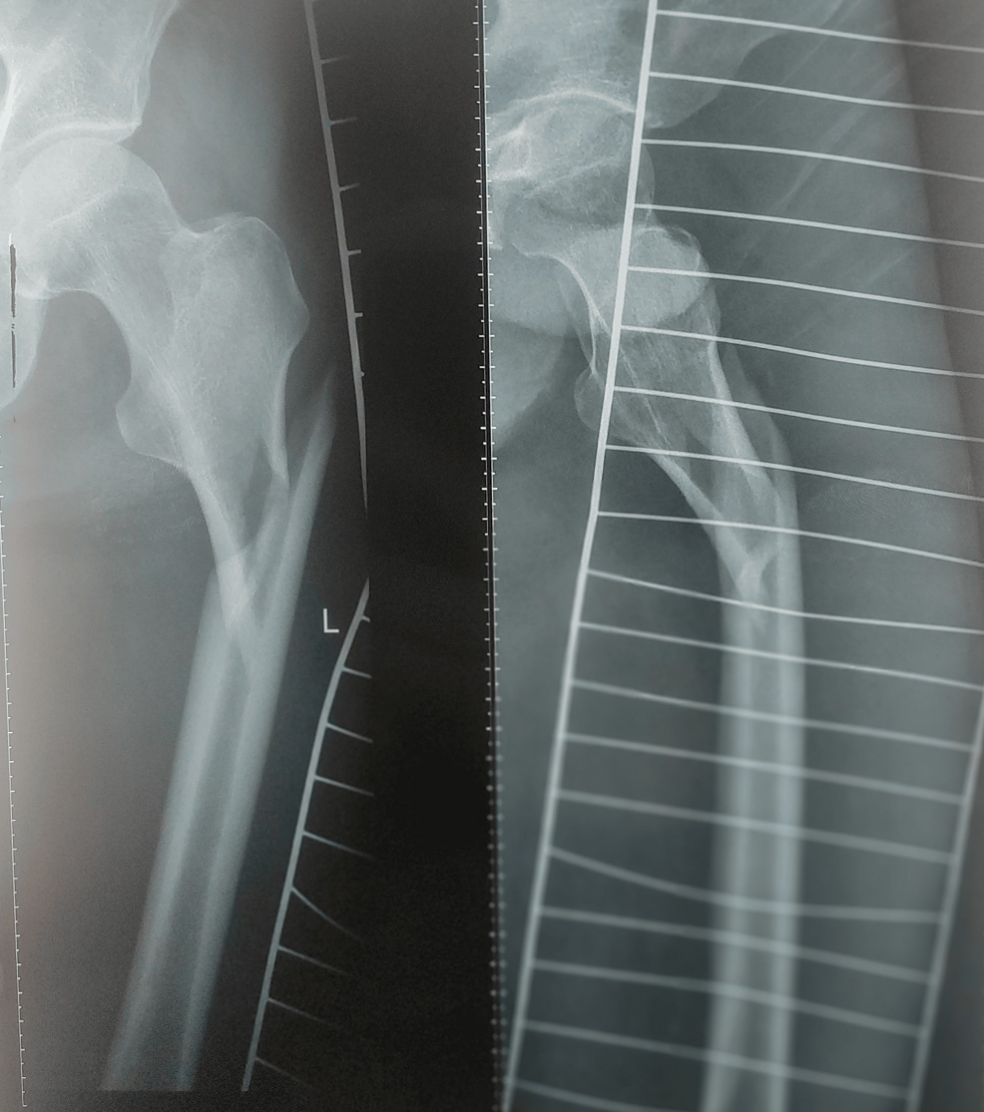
Preoperative X-ray (lateral view) showing the subtrochanteric fracture
